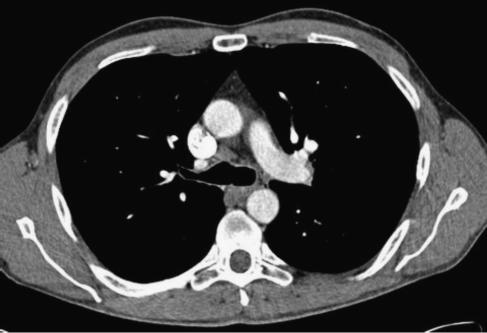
Precarinal lymph nodes 1cm in size, unchanged on sequential chest CT.
